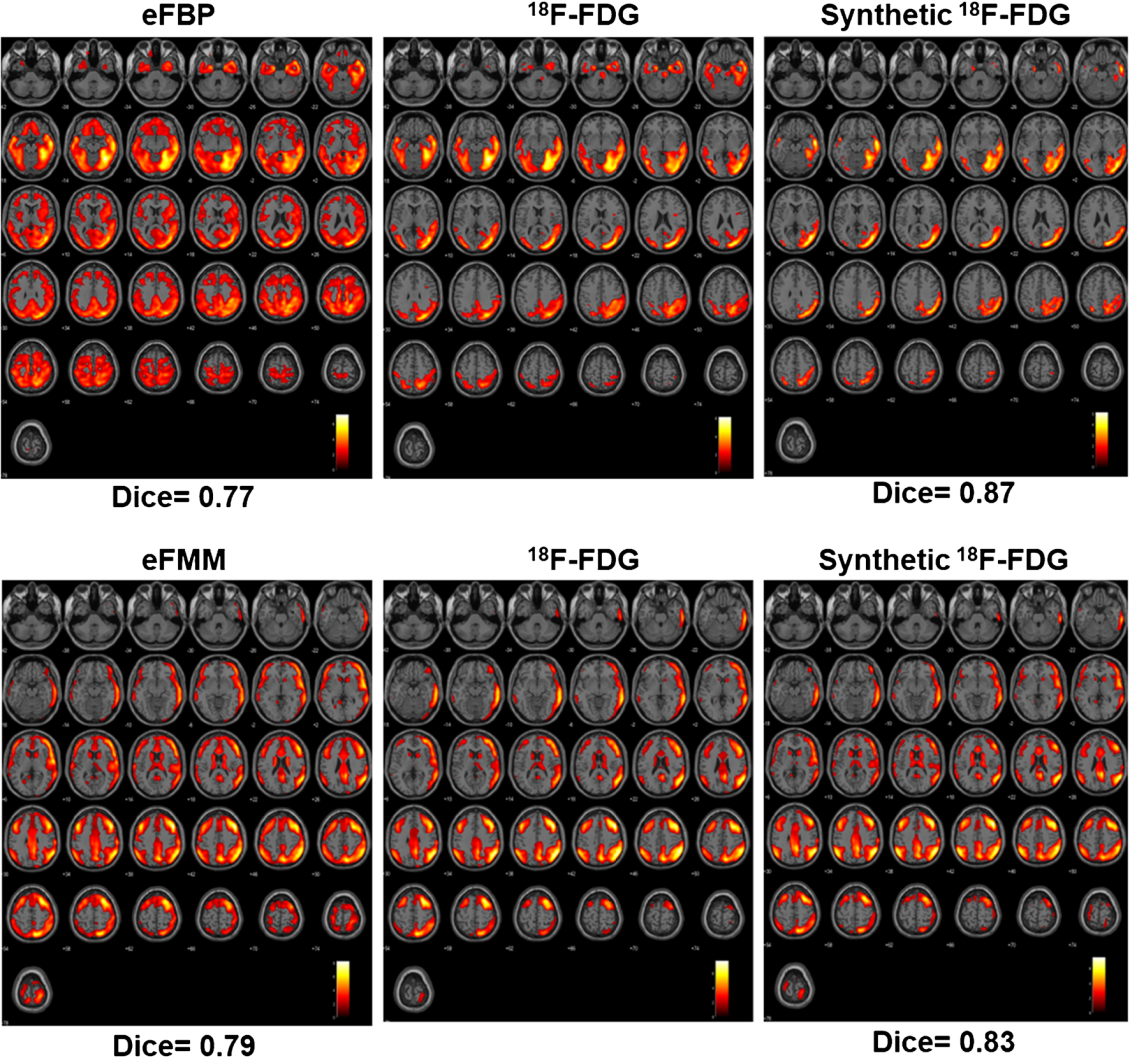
The SPM-t maps of hypoperfusion/hypometabolism of two single cases of AD patients for the two tracers (eFMM/eFBP), shown as example. All t-maps for each case were produced compared to the same set of [^18^F]FDG PET scans in the healthy control subjects. Yellow/red scales shown in SPM maps are regions which are hypoperfused/hypometabolic in these patients in comparison to the normal control database (see text for details)
